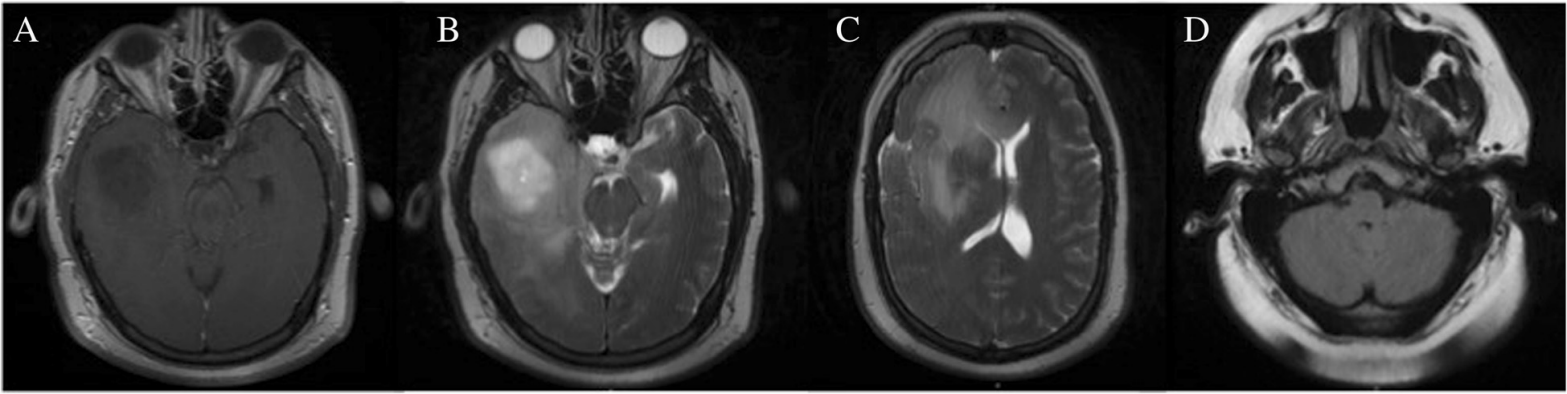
Imaging features of the initial tumor. a T1-weighted post-contrast and b T2-weighted MRI demonstrated a non-enhancing lesion in the right temporal lobe. Edema extended superiorly on T2-weighted MRI (c) to involve the fronto-parietal lobes and corpus callosum. d T2-weighted FLAIR MRI did not reveal any disease in the cerebellum
